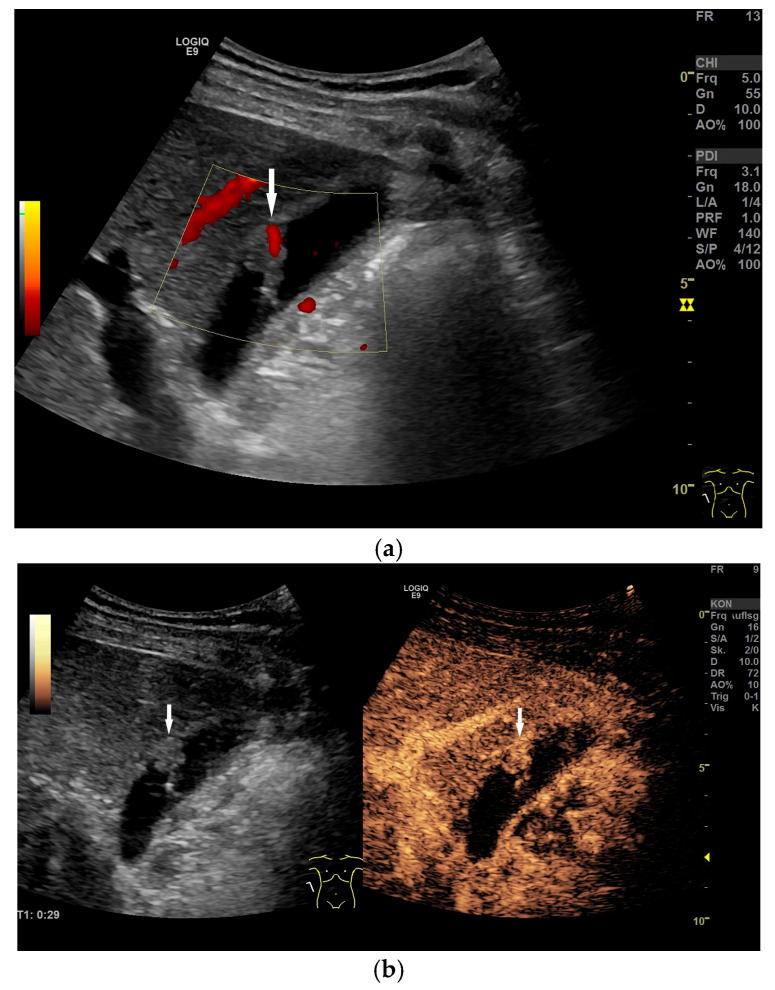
GB polyp: Irregularly shaped polyp of maximal size of 17 mm with a central vessel (arrow) on power Doppler imaging (a), proving that the finding is not an artifact. On CEUS, the polyp is enhanced (arrow) (b), excluding sludge. Cholecystectomy is indicated. (Source: own collection, K.M.).
